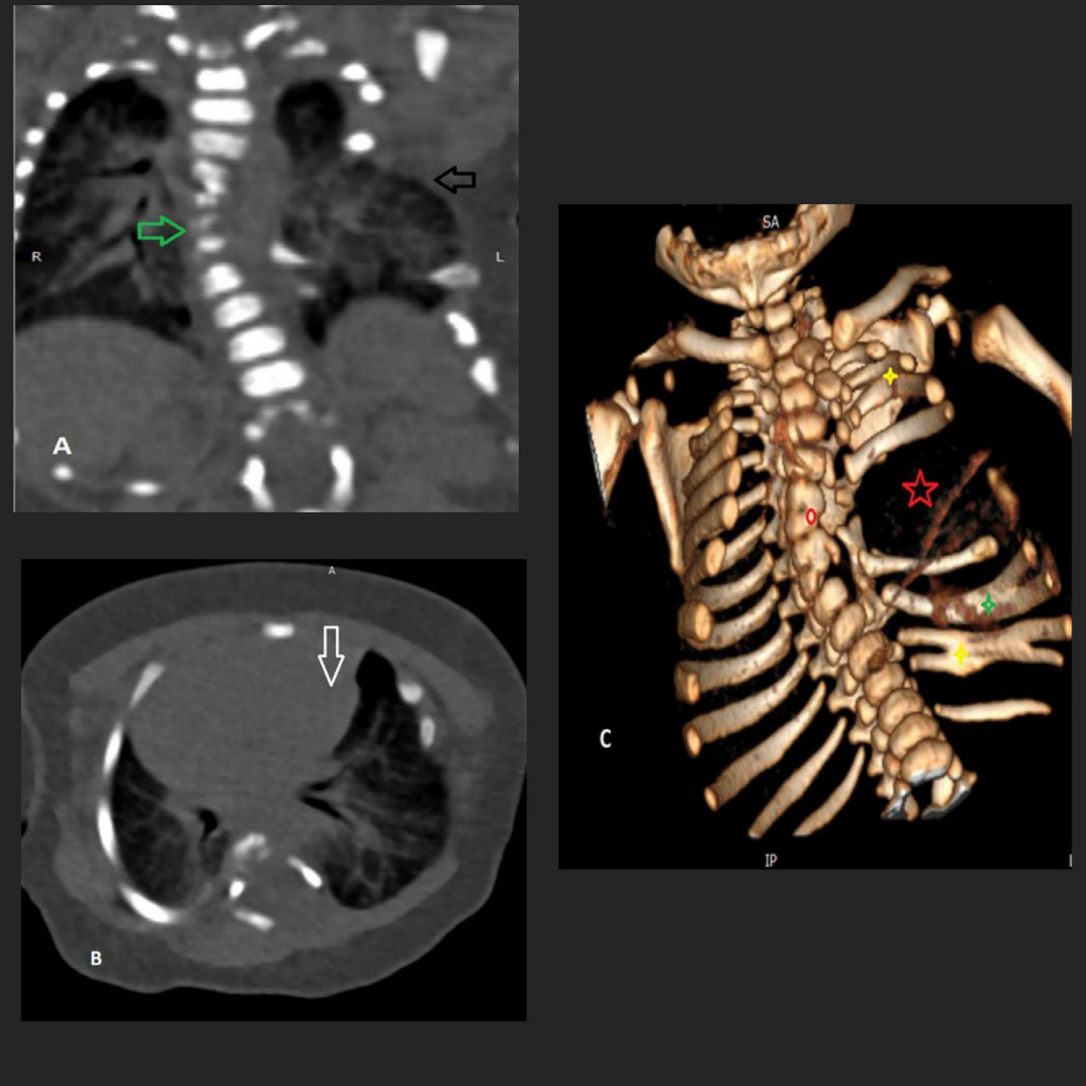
Chest CT scan findings. (A) Coronal view, (B) axial view, and (C) 3D reconstruction confirm absence of the left 5th, 6th, and 7th ribs (red star). Partial fusion is observed in the mid-segments of the 2nd and 3rd ribs and the 10th and 11th ribs (yellow star). The left 9th rib appears bifid (green star). The heart is dextropositioned (white arrow), and herniation of the left lung through the rib defect is evident (black arrow). A left-sided vertebral bar at the T7-T8 level is noted, as well as a hypoplastic butterfly vertebra at T6. Mild levoscoliosis is present in the mid-thoracic spine (red circle).
